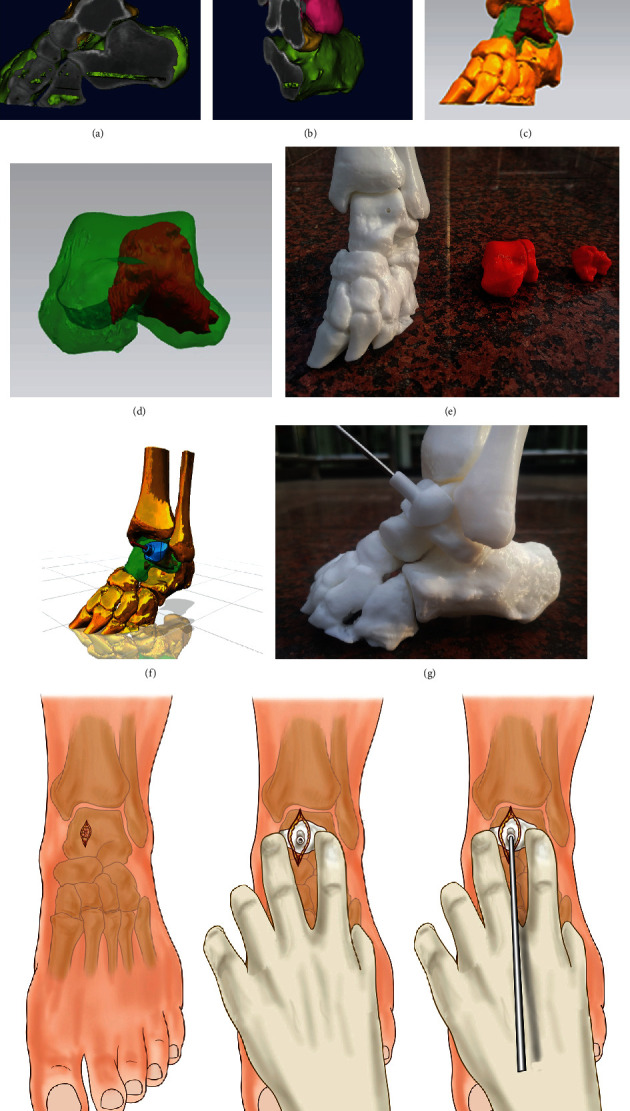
Preoperative preparation of 3D-printed lesion models and individualized guide system. (a, b) Talar lesions established based on the 3D CT images before surgery; (c, d) the software was used to distinguish lesions from healthy tissues; (e) 3D-printed lesion model that could be used for surgical planning and rehearsal; (f, g) design and preparation of individualized guides to assist in determining the position of the Kirschner wire. (h) The location of the lesion was entered through the incision, and the bony structure was confirmed; (i) the anatomical position was determined through the bony structure, and the cyst position was located with the guide; (j) the guide was used to assist the accurate positioning of the Kirschner wire.
